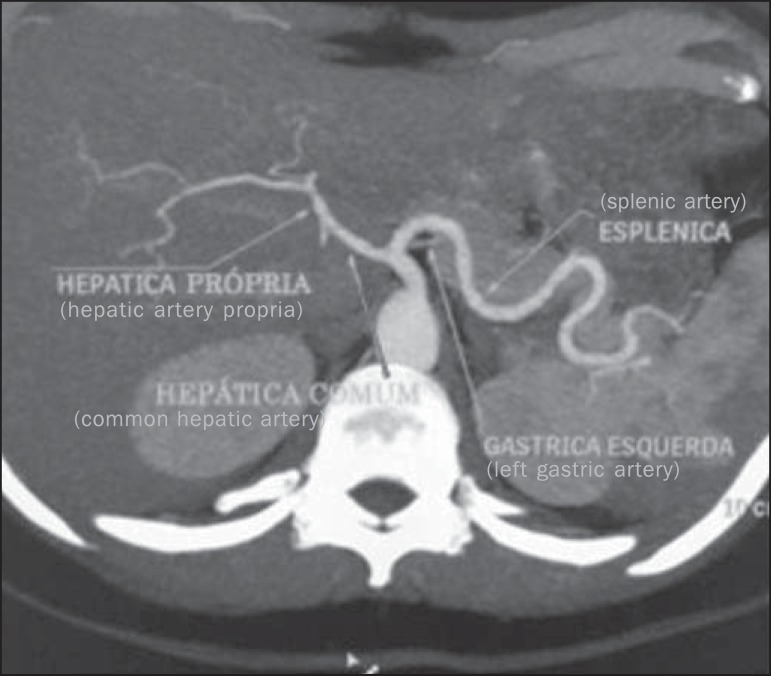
Contrast-enhanced axial CT demonstrating a normal CAT. The celiac artery
